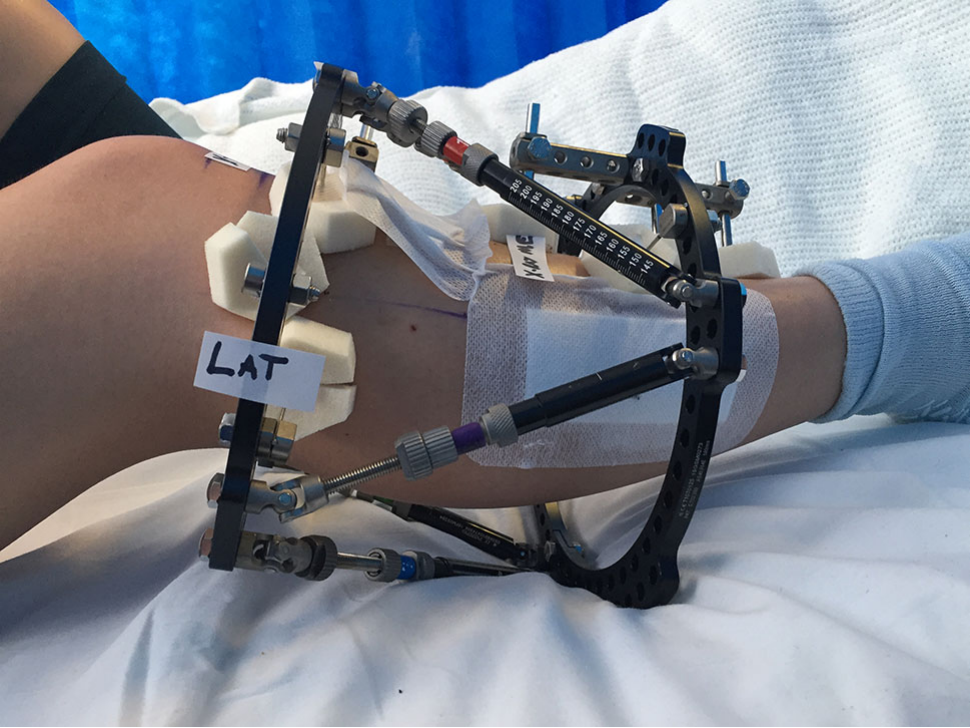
Post-operative photograph demonstrating positioning of “lateral” sticker indicating centre of X-ray beam
